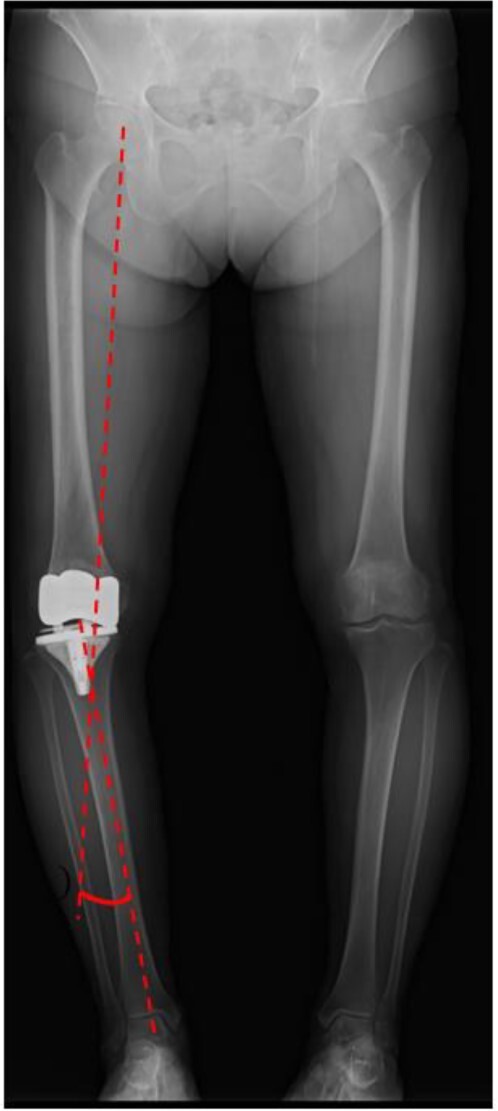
Post-operative standing plain radiograph. The hip-knee-ankle angle was 14° varus.
